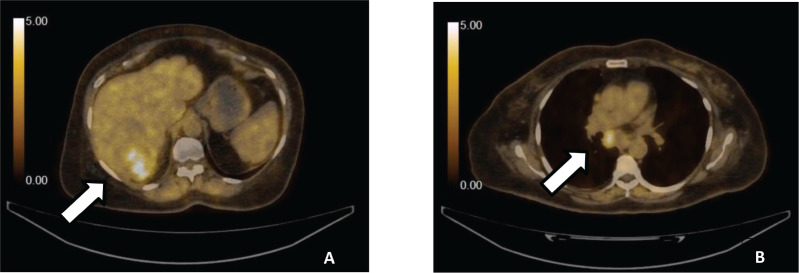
(a): PET-CT demonstrating liver with cirrhotic changes with low FDG avid necrotic hypodense lesion seen involving the right lobe. (b): Metastatic hepatocellular carcinoma showing low FDG avid subcarinal nodes.
